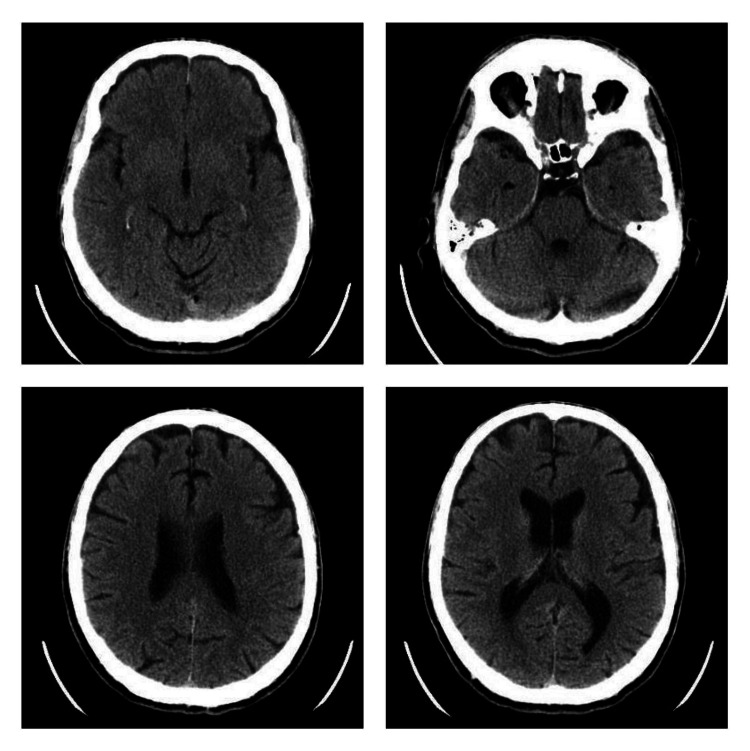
Images from the brain CT scan of the patient demonstrating no abnormalities CT: computed tomography
